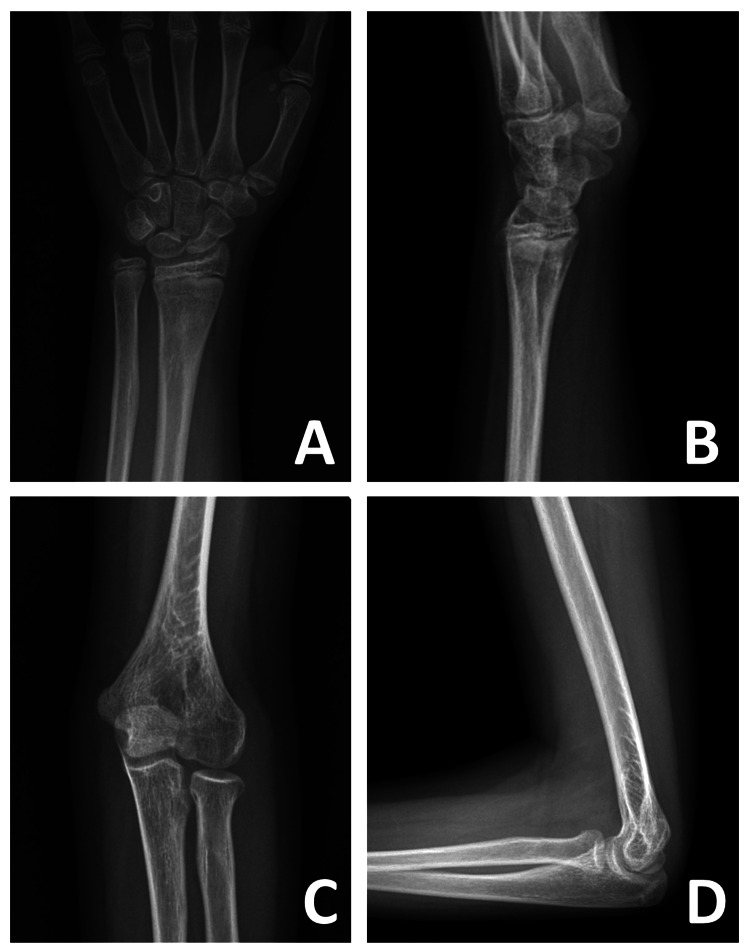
Radiographs taken at one-year postoperative follow-up (A,B) Anteroposterior and lateral radiographs of the left wrist. (C,D) Anteroposterior and lateral radiographs of the left elbow.
